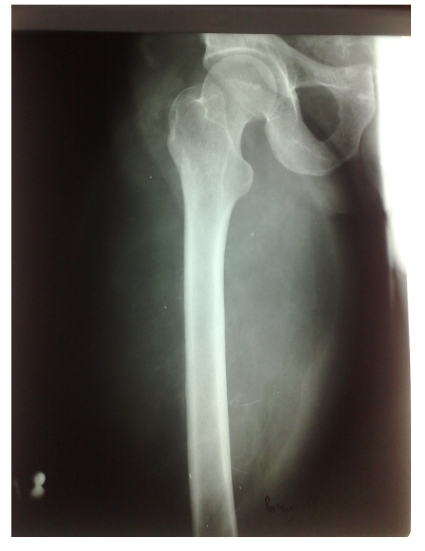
Radiography of right thigh
